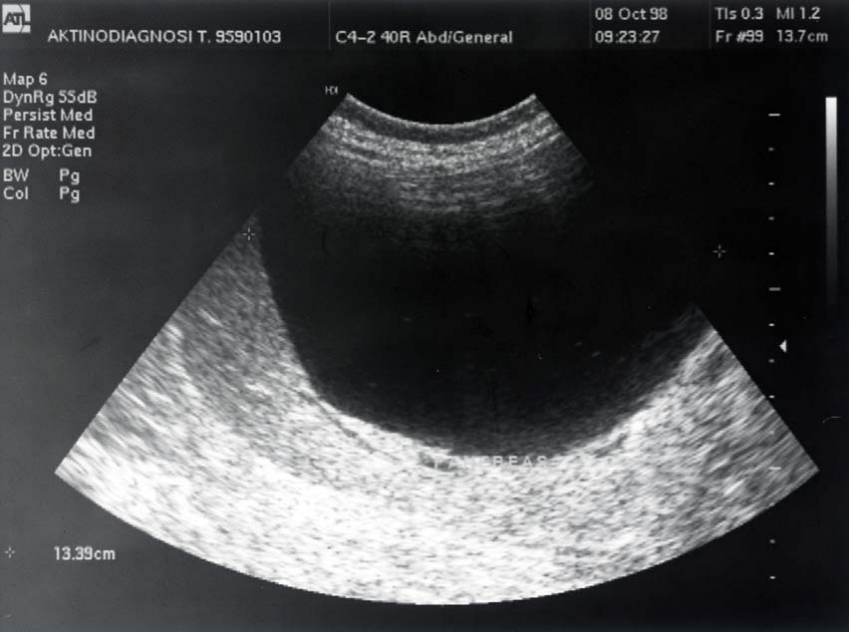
Another view via ultrasonography of the patient's simple liver cysts two years before his latest admission.
